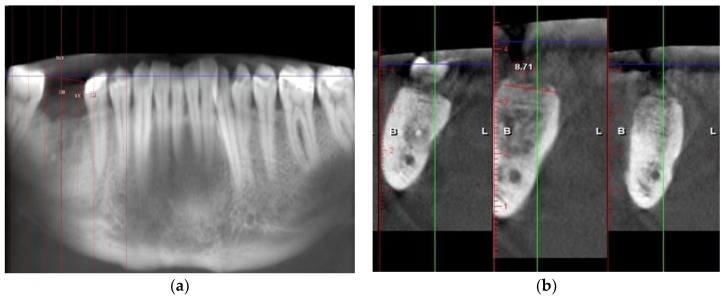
Radiographic examination and measurements in (a) vertical and (b) horizontal dimension.
